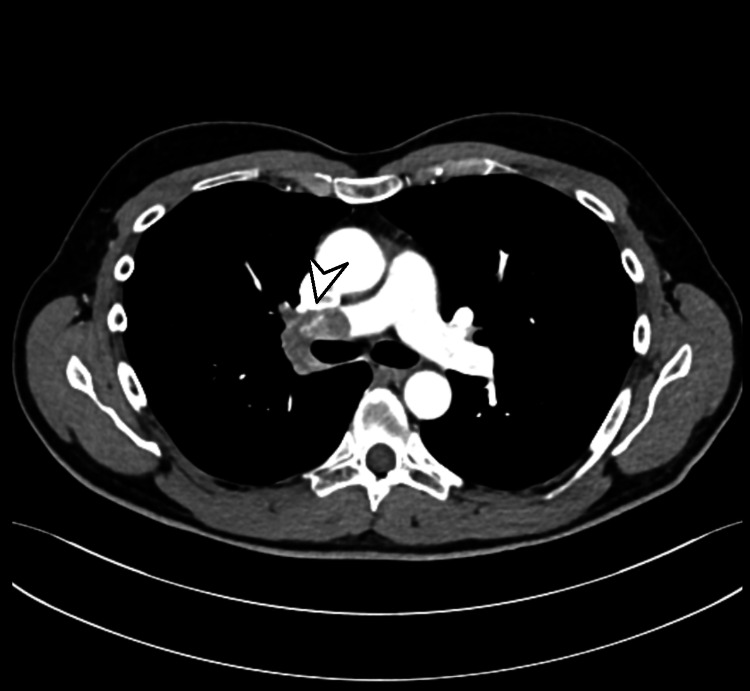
Chest CT showing infiltration of the main neoplastic lesion into the right pulmonary artery (white arrowhead) CT: computed tomography
